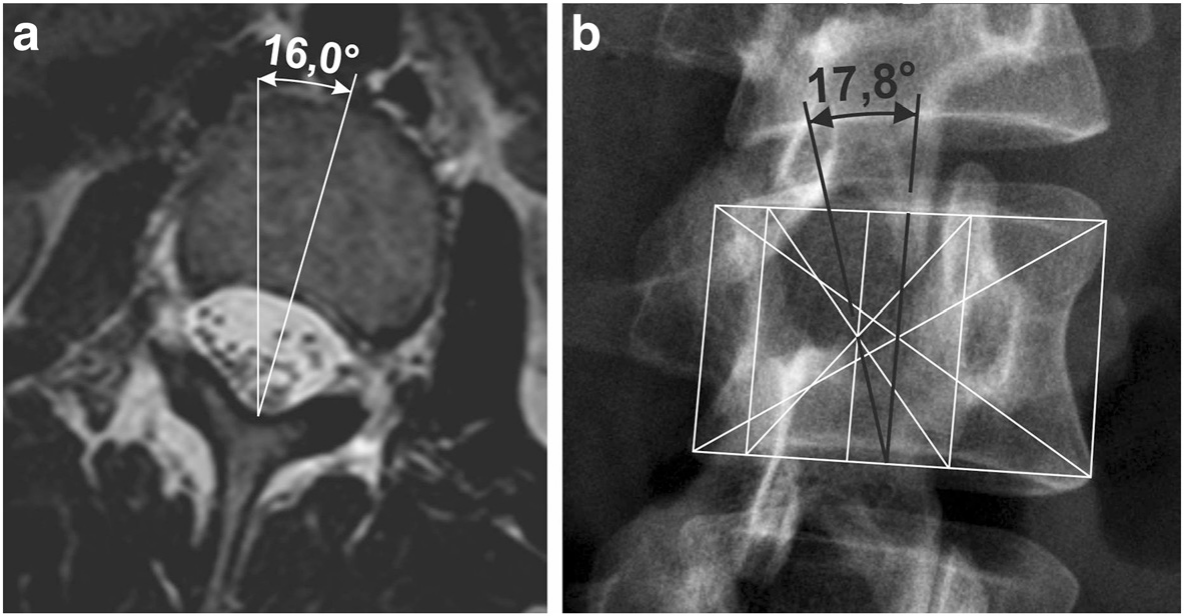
Comparison of vertebral rotation measurement. MRI picture in transversal plane (a). Evaluation of axial vertebral rotation by the new radiographic method - table 3, case 2, vertebra L2 (b).
